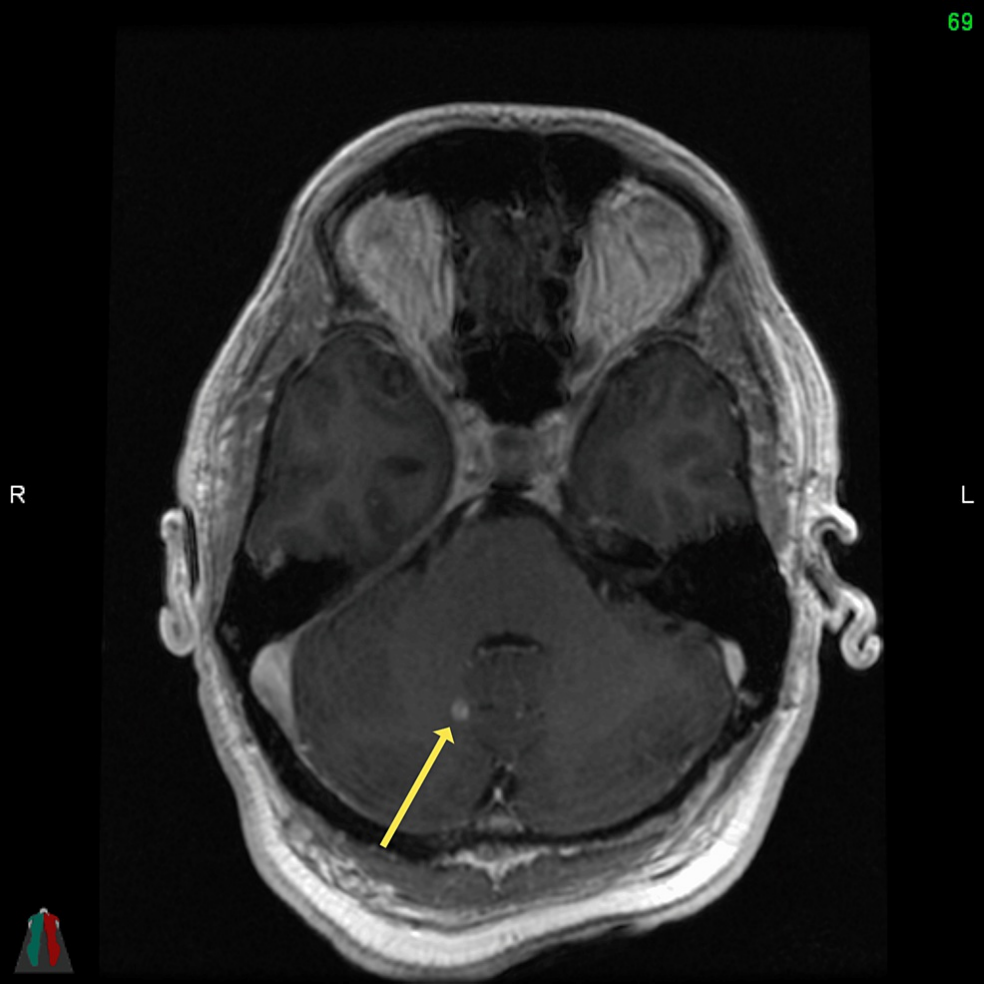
Axial head MRI T1 FSPGR post-contrast demonstrating right cerebellar hemisphere nodule measuring 5 mm in keeping with metastatic deposit FSPGR: fast spoiled gradient-echo
